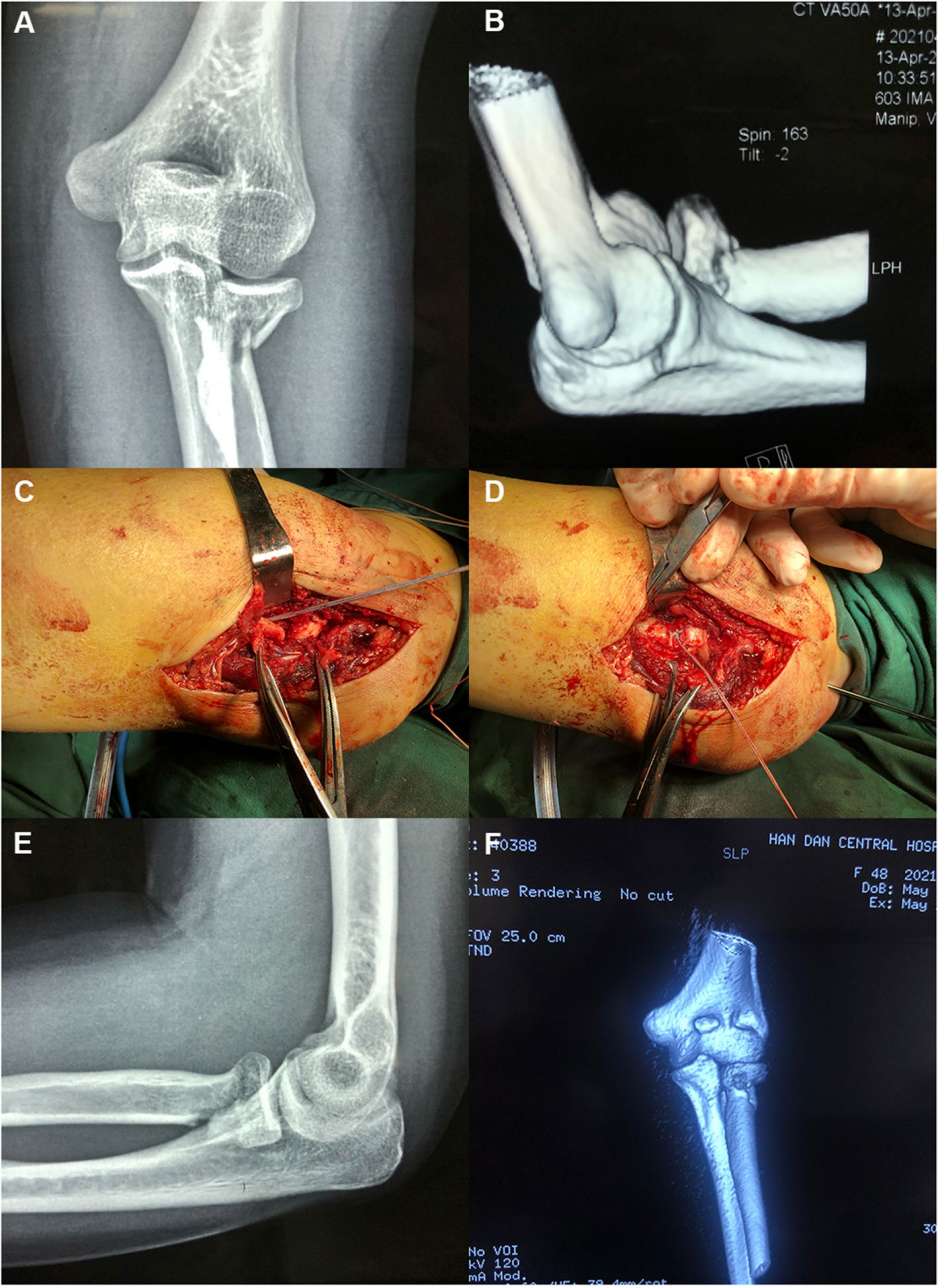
Case 1: Preoperative X-ray (A) and spiral CT (B) reveal a type III fracture of the left radial head and neck with displacement. During the operation, there is splitting displacement in the junction area of the radial head and neck (C), and the articular cartilage at the edge of the radial head is comminuted (C, thick arrow). An all-suture anchor (4.5 mm) is implanted into the bone cortex at the distal end of the fracture from inside to outside (C, fine arrow). The proximal end of the tail thread of the anchor is folded in a T shape and runs through the osteochondral injury site at the edge of the radial head to the opposite side. After the reduction is satisfactory, the tail thread of the suture anchor is tightened at the neck of the radius and knotted (D, thick arrow). A postoperative X-ray (E) and CT scan (F) revealed that the alignment of the RHF is good
